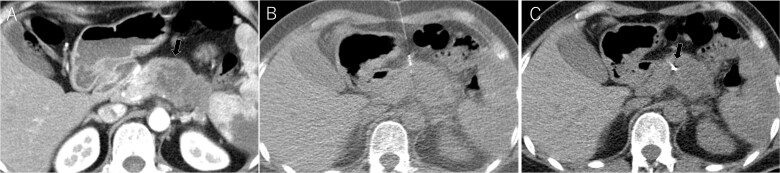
A 53-year-old male with pancreatic cancer. (A) Contrast-enhanced CT shows pancreatic cancer at the pancreatic body and tail (arrow). (B) Percutaneous fiducial marker placement was performed. The needle was inserted through the ventral side of the pancreatic cancer. (C) Postprocedural CT shows an implanted fiducial marker beside the pancreatic cancer without migration (arrow).
